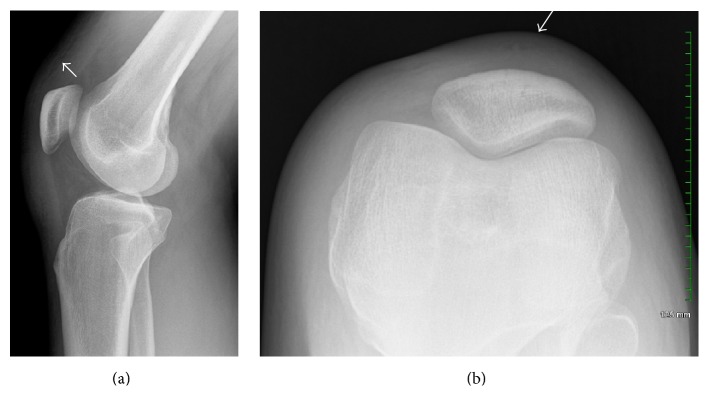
Plain radiography of the right knee. Lateral (a) and sunrise (b) views revealed anterior soft tissue swelling particularly in the superomedial patellar region (arrows).
